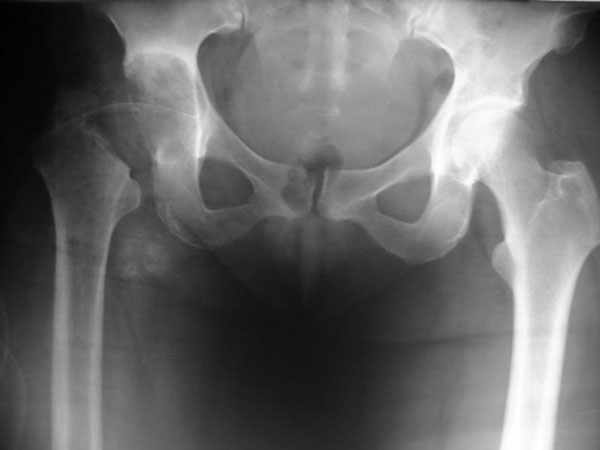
Hip x-ray showing severe desctruction of periarticular bony destruction and deformity of the right hip.
